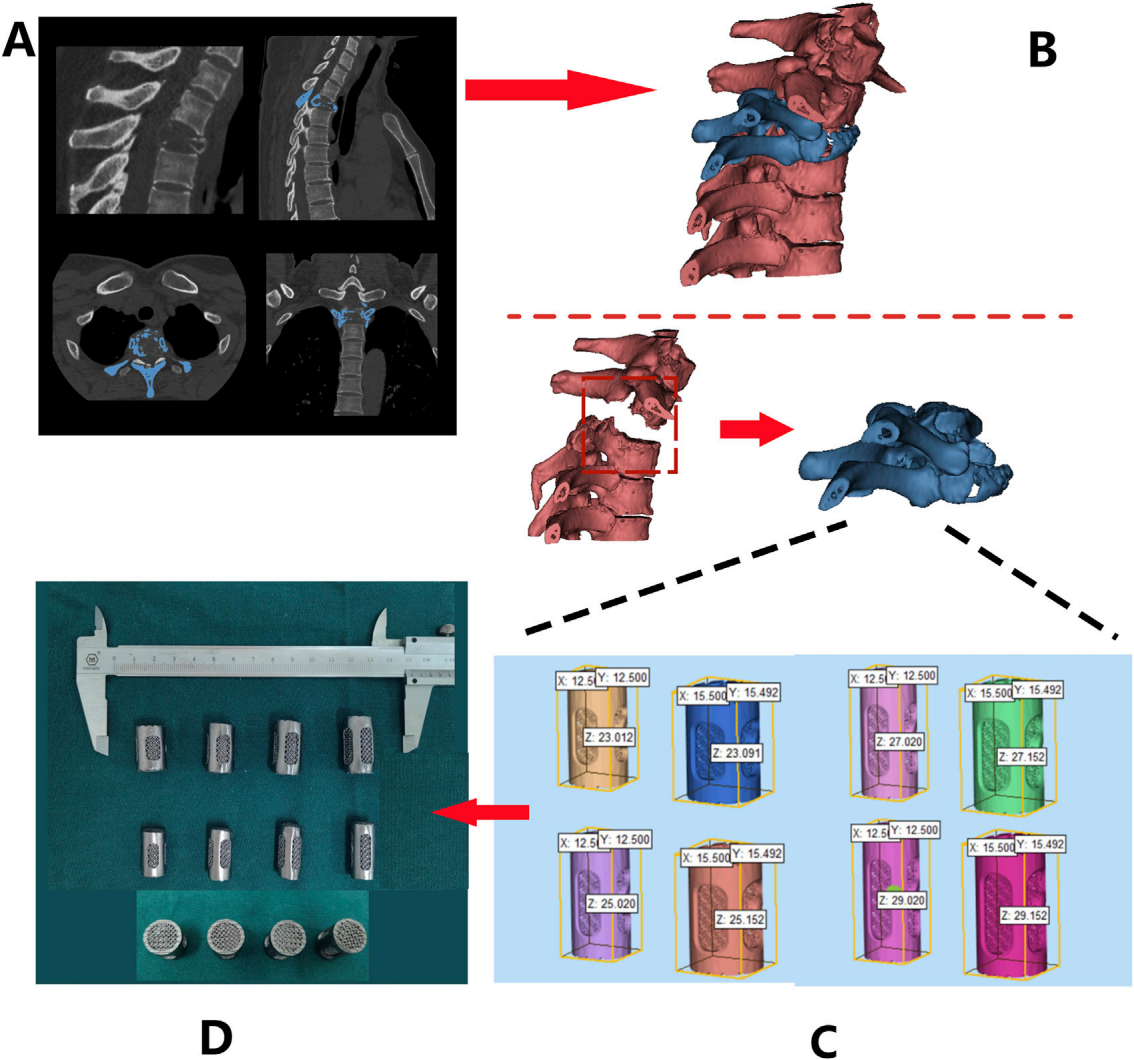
Design and fabrication process of 3D-printed porous tantalum vertebral body fusion implants (Patient 1 as an example): (A) data acquisition via thin-slice CT scanning; (B) three-dimensional reconstruction using MIMICS software based on CT data to generate a 3D model for analyzing lesion sites and other conditions; (C) implant design based on the 3D model; (D) final fabrication of implants.
